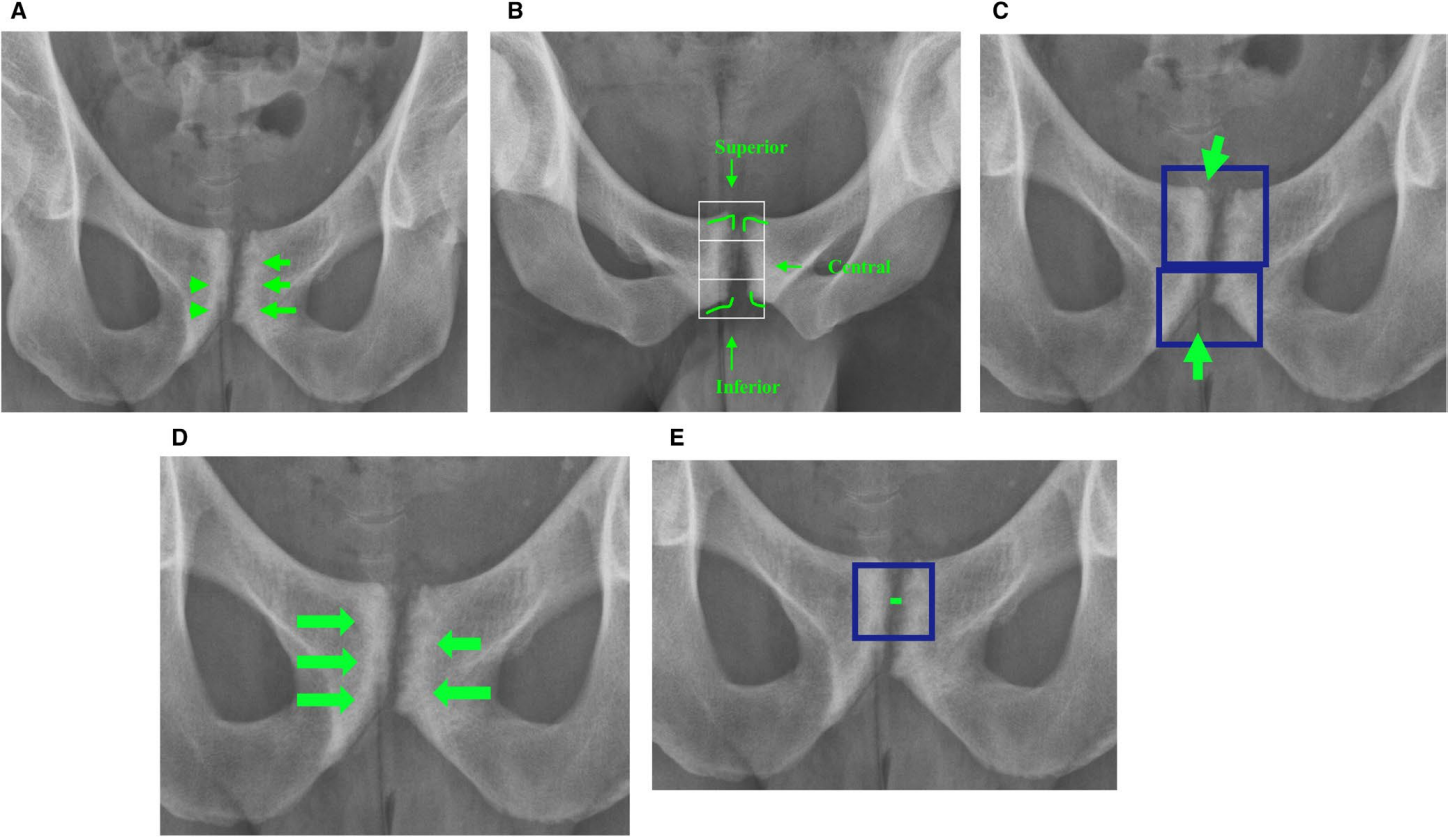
Pubic‐related radiographic findings as described by Serner et al. [22].^.^ (A) Bone lucency. Definition: “A clear area of decreased attenuation compared to the surrounding bone, which corresponds to an erosion‐like configuration and/or cyst.” [22], Subclassifications: *Erosion‐Like Configuration (ELC), Superior/Central ELC, Inferior ELC and Cysts*. Definitions: *ELC*: “Irregularities of the cortical bone surface, potentially accompanied by loss of the adjacent trabecular bone.” [22], *Superior/Central ELC*: ELC at the superior two thirds of the joint surface [22], *Inferior ELC*: ELC at the lower third of the joint surface ‐ “If the entire lower half was considered to have an erosion‐like configuration, both of the above were scored as positive” [22], *Cysts*: “Areas of bone lucency with a sclerotic rim inside the trabecular bone compartment, without accompanying cortical bone surface irregularity.” (B) Proliferation. Definition: “Clear osteophyte outgrowths at the joint margins or within the articular space.” [22], Subclassifications: *Superior, Central and Inferior Proliferation*. Definitions: *Superior proliferation*: “This can be considered “bone spurs” or classified as “pubic beaking” when bilateral. Well rounded (smooth) bumps at the superior aspect, even if asymmetrical in size, were not considered proliferation. For superior proliferation, the “sharpness” of the superior bone corner angle was used for assistance with angles higher than 90 deg. (obtuse angle) considered “rounded” and scored negative/absent, whereas angles lower than 90 deg. (acute angle) were considered “sharp” and scored as positive/present.” [22], *Central proliferation*: “Proliferation at the central portion of the articular space.” [22], *Inferior proliferation*: “Similar considerations as superior proliferation.” [22]. (C) Fragmentation. Definition: “Clear loose fragment(s) within the symphyseal joint space, or at the inferior medial margin of the pubic bone.” [22], Subclassifications: *Central and inferior fragmentation*. Definitions: *Central fragmentation*: “Clear loose fragment(s) within the symphyseal joint space” [22], *Inferior fragmentation*: “Clear loose fragment(s) at the inferior medial margin of the pubic bone.” [22]. (D) Sclerosis. Definition: “A clear area of increased attenuation of the subchondral bone compared to the surrounding bone, corresponding to an area of increased bone density.” [22]. (E) Pubic Symphysis Joint Space Width. Definition: “Symphyseal joint space measured in millimeters at the narrowest point of the joint surfaces.” [22], Subclassification: *Narrow Joint Space Width*. Definition: *Narrow Joint Space Width*. “if measured to less than 3mm” [22].
